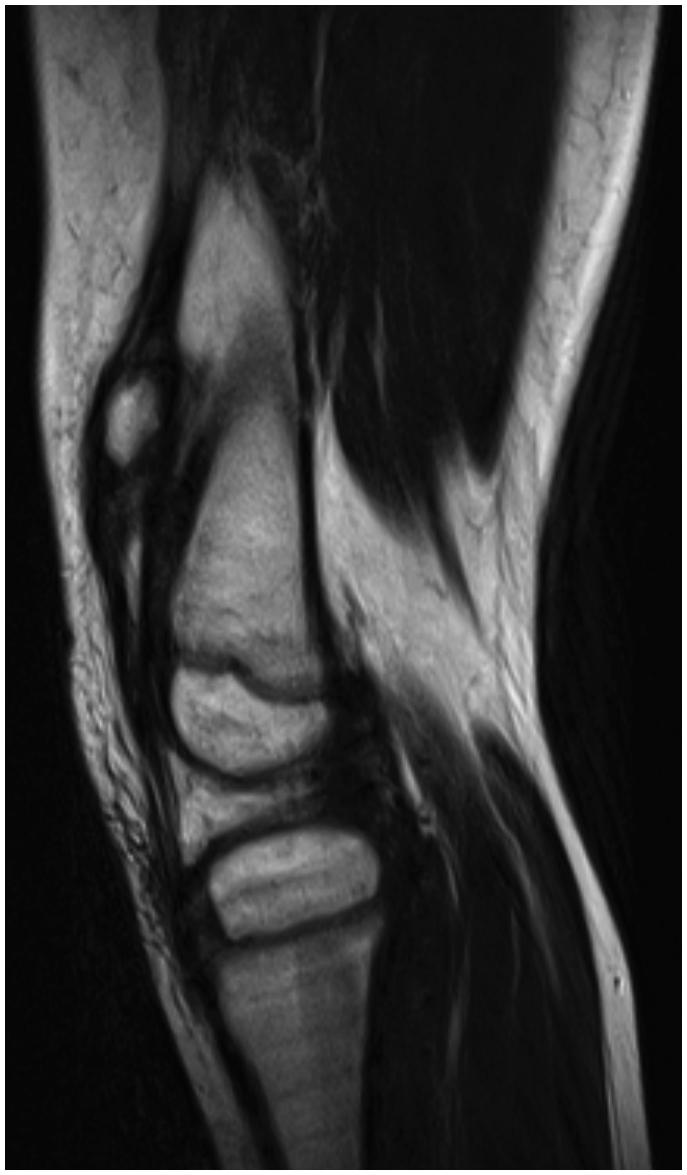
T2 weighted MRI image of the left knee. The patella is proximally located. The ligamentum patella is seen with normal thickness and signal intensity in the most proximal part, but is ruptured distally.
